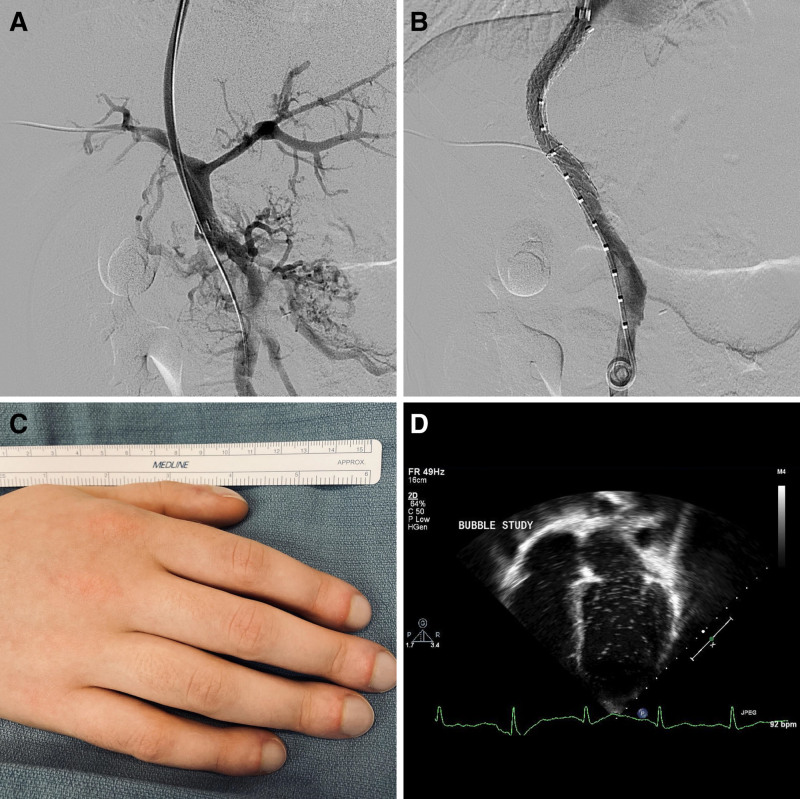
Frontal projection digital subtraction portal venography before (A) and after (B) creation of a transjugular intrahepatic portosystemic shunt. Photograph of the hand (C) demonstrated digital clubbing. Echocardiography with bubble study (D) demonstrated appearance of microbubbles within the left cardiac chambers at 7 cardiac cycles after systemic infusion, confirming intrapulmonary shunting, and the diagnosis of hepatopulmonary syndrome.
